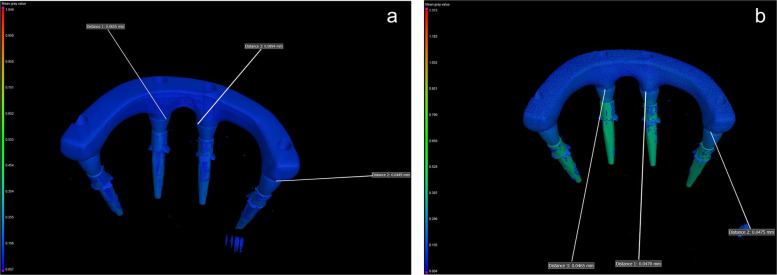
Color difference material X-ray attenuation maps of 3-dimensional (3D) marginal discrepancy values measured at different locations of abutment-framework interface (mesial, buccal, lingual, distal) for each implant. A, Milled titanium framework. B, Additively manufactured titanium framework.
